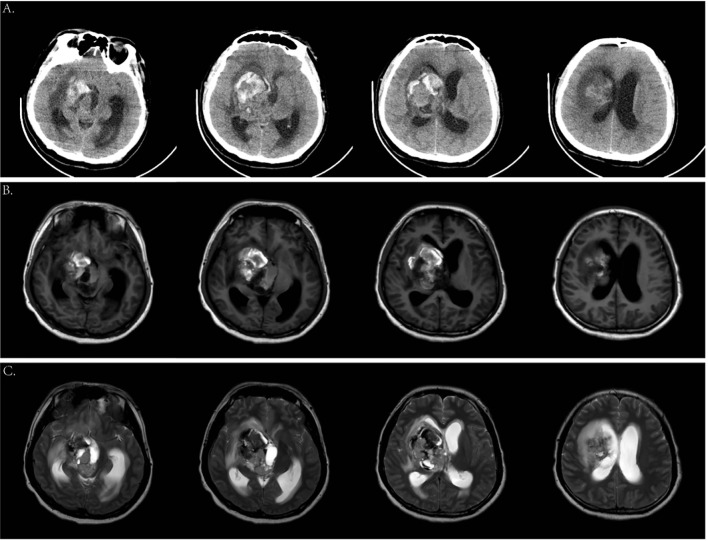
Cranial computed tomography (CT) scan and magnetic resonance imaging (MRI) on the current admission. (A) Emergency head CT scan showed an amount of hemorrhage in the right basal ganglia. (B) Axial T1-weighted images. (C) Axial T2-weighted images.
